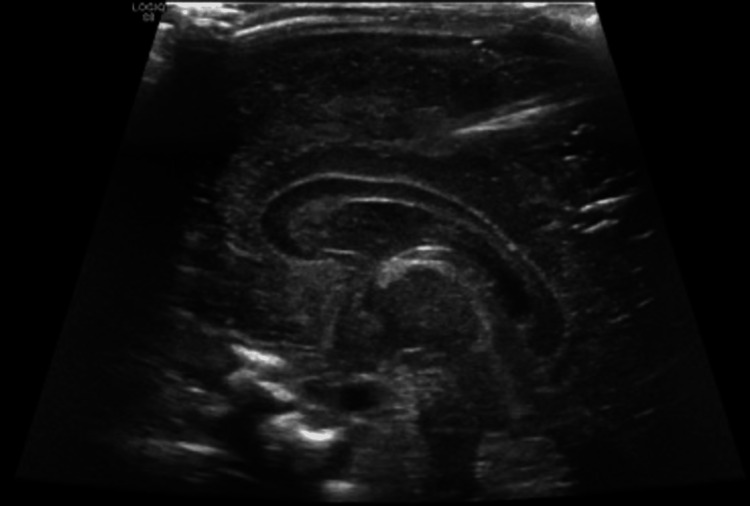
Transcranial ultrasound (sagittal view) showing diffuse hypoechoic appearance of the brain parenchyma with indistinct gray-white matter interface and symmetrical ventricular system attenuation. Scattered innumerable hypoechogenic foci. No signs of intracranial hemorrhage.
